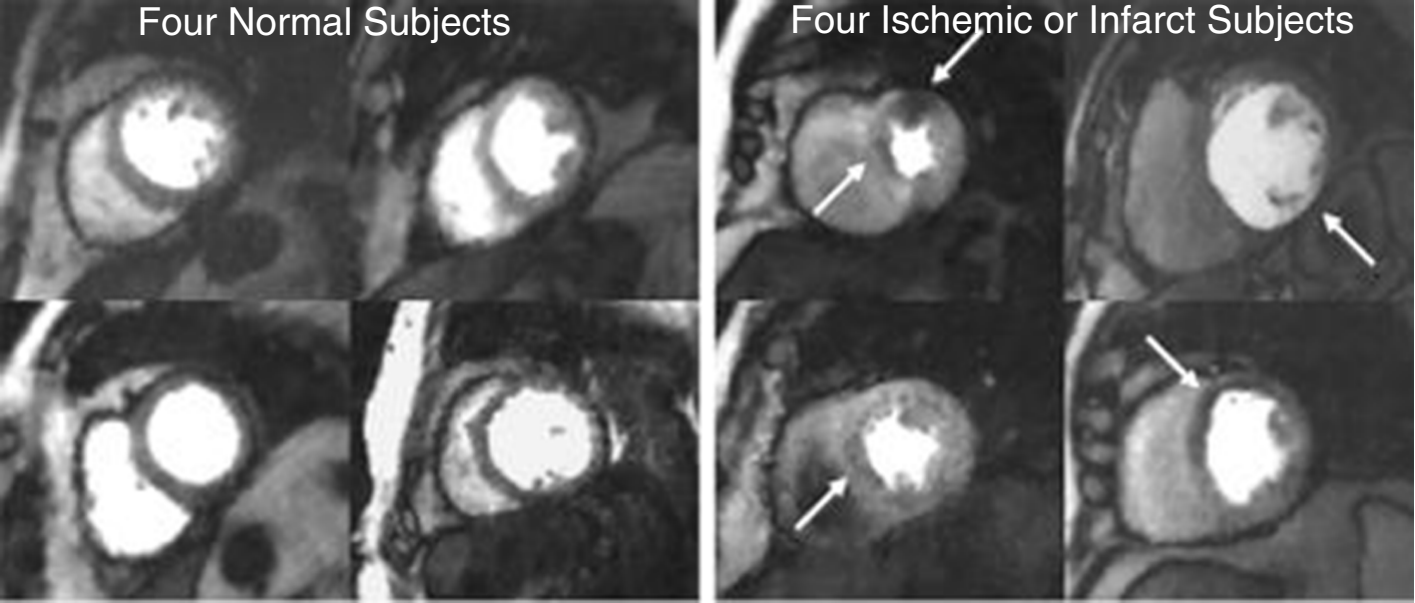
One time frame of one slice from each of the 8 patients - ungated stress perfusion imaging. The four images on the left are from patients with normal x-ray angiography results. The four images on the right depict reversible ischemia in two images and fixed perfusion defects consistent with infarction in the far right two images.
